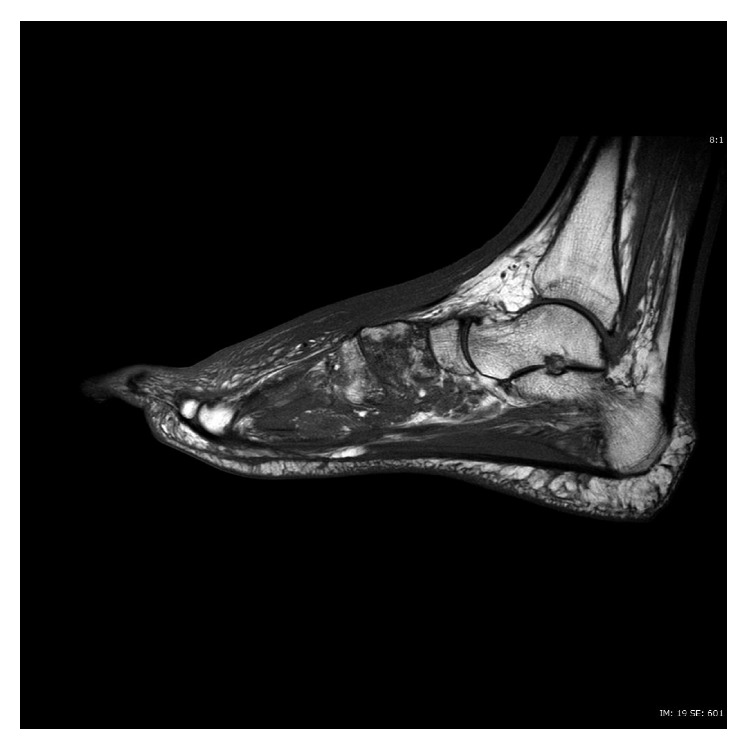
T1-weighted sagittal image of MRI of left foot without contrast, showing medullary edema and osteomyelitis of cuneiforms and metatarsals.
